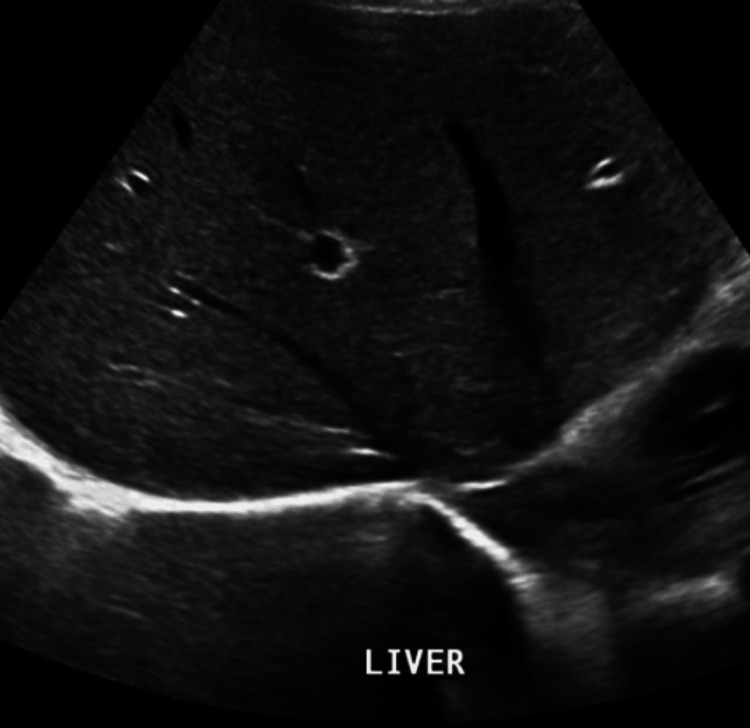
Abdominal ultrasound revealed an unremarkable study except for fatty liver changes.
